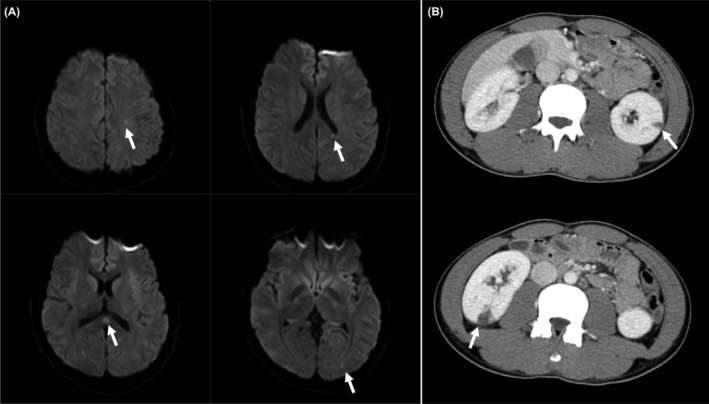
(A) Head magnetic resonance imaging showing multiple cerebral infarcts (arrows). (B) Enhanced computed tomography of the abdomen showing multiple renal infarcts in the bilateral kidneys (arrows).
